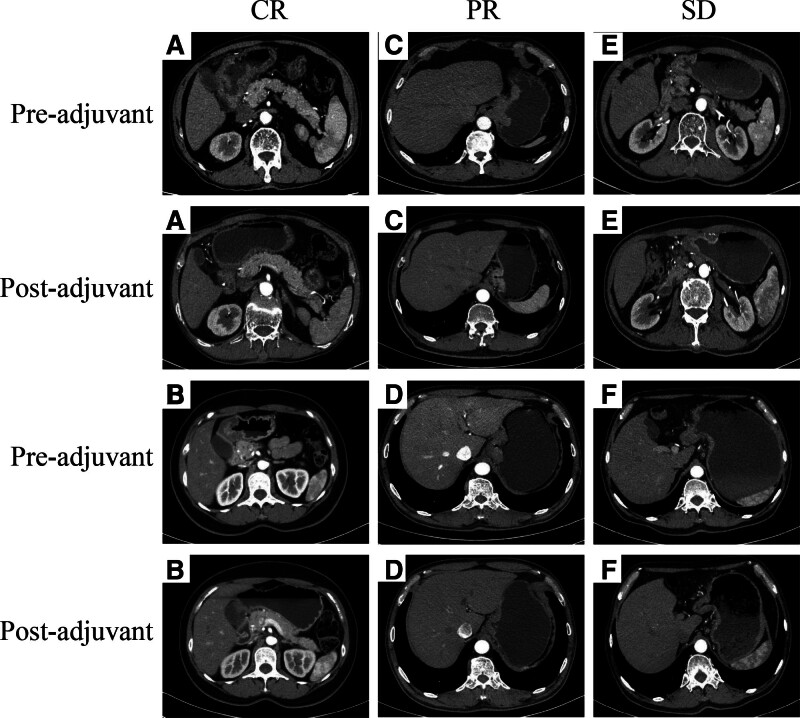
CT scan of the standard patients who achieved CR, PR, and SD after 2 to 3 cycles before and after neoadjuvant treatment. CR = complete response, CT = computed tomography, PR = partial response, SD = stable disease.
